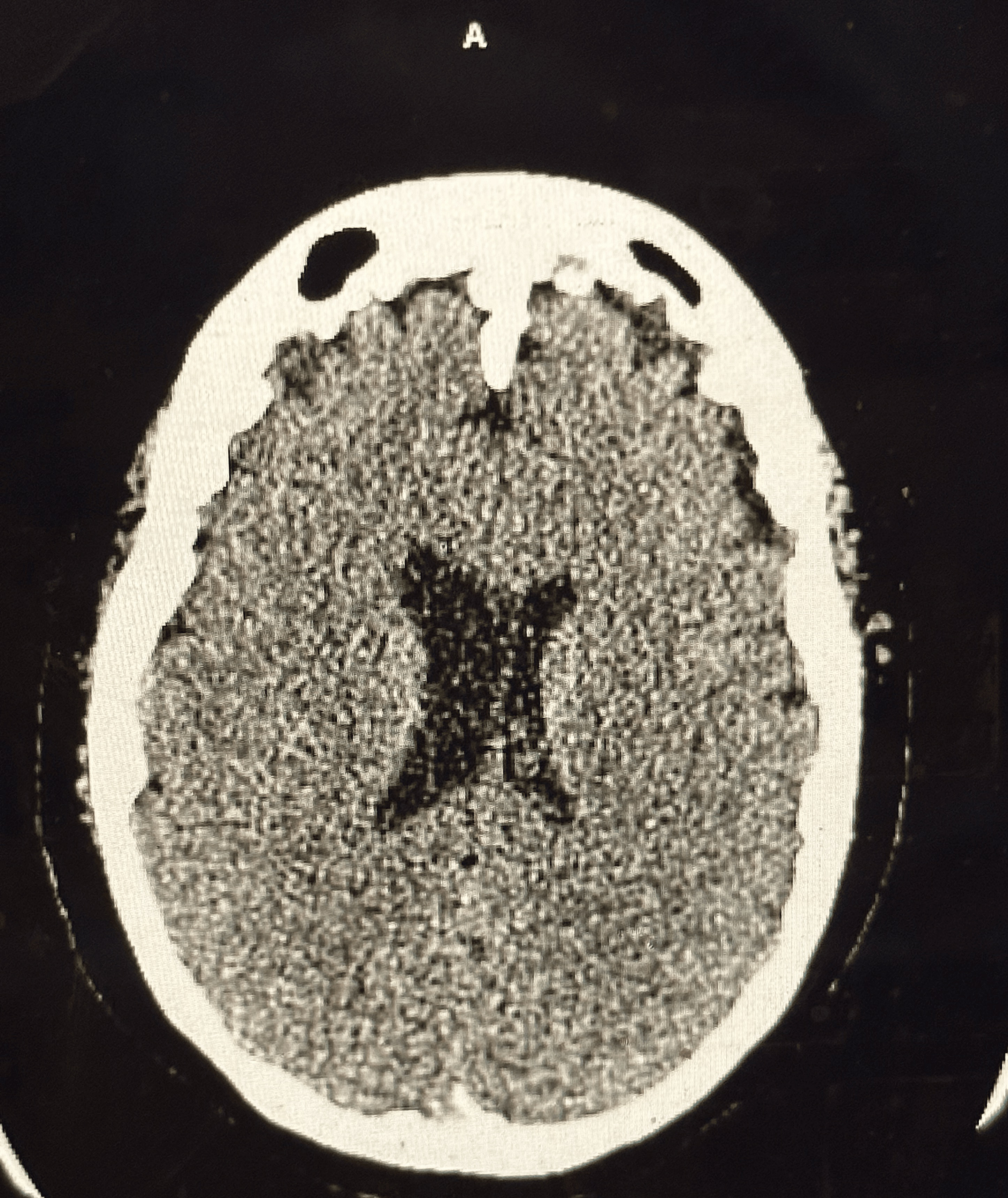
CT scan of head or brain without contrast This image shows no evidence of acute abnormality as a cause of generalized weakness, tingling, and numbness. Ventricles and sulci are normal in size and shape. Ventricular asymmetry is noted, which is a normal variant.
